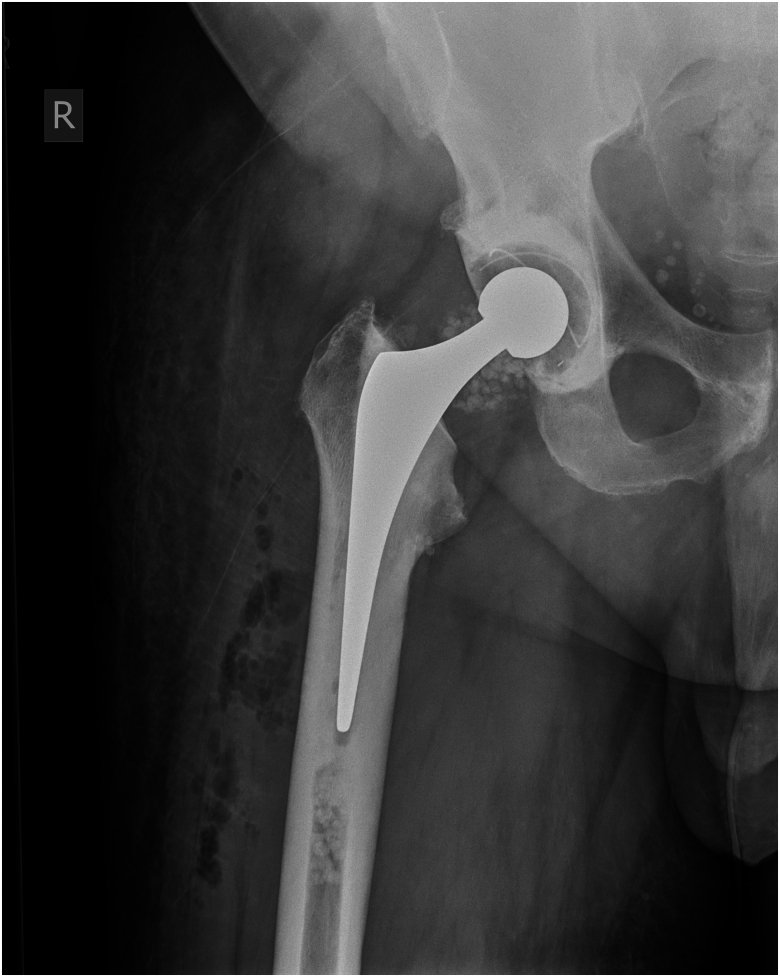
Revision total hip replacement immediately post surgery. The anteroposterior x-ray of the hip demonstrates the antibiotic-loaded calcium sulfate beads acting as an effective cement restrictor in the medullary canal.
